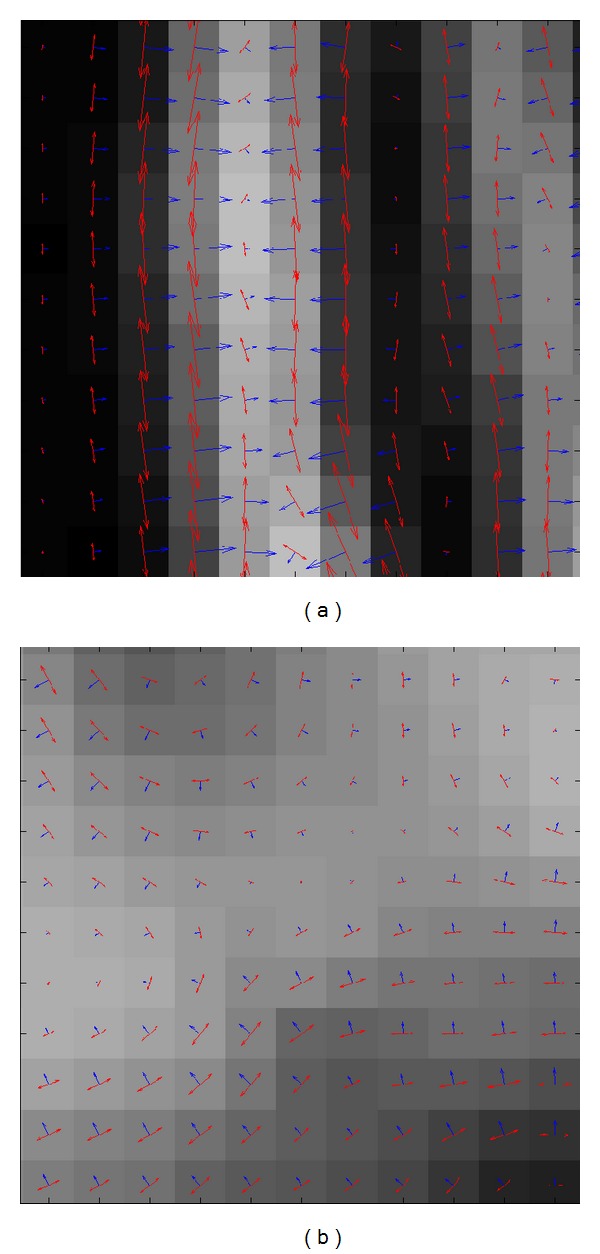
Local orientations of patterns in T1 MRI (Figure 2(a)). (a) Straight pattern. (b) Curved pattern. Note that the blue arrow shows the local gradient orientation at each pixel, and the length of the blue arrow denotes the value of local gradient magnitude.
